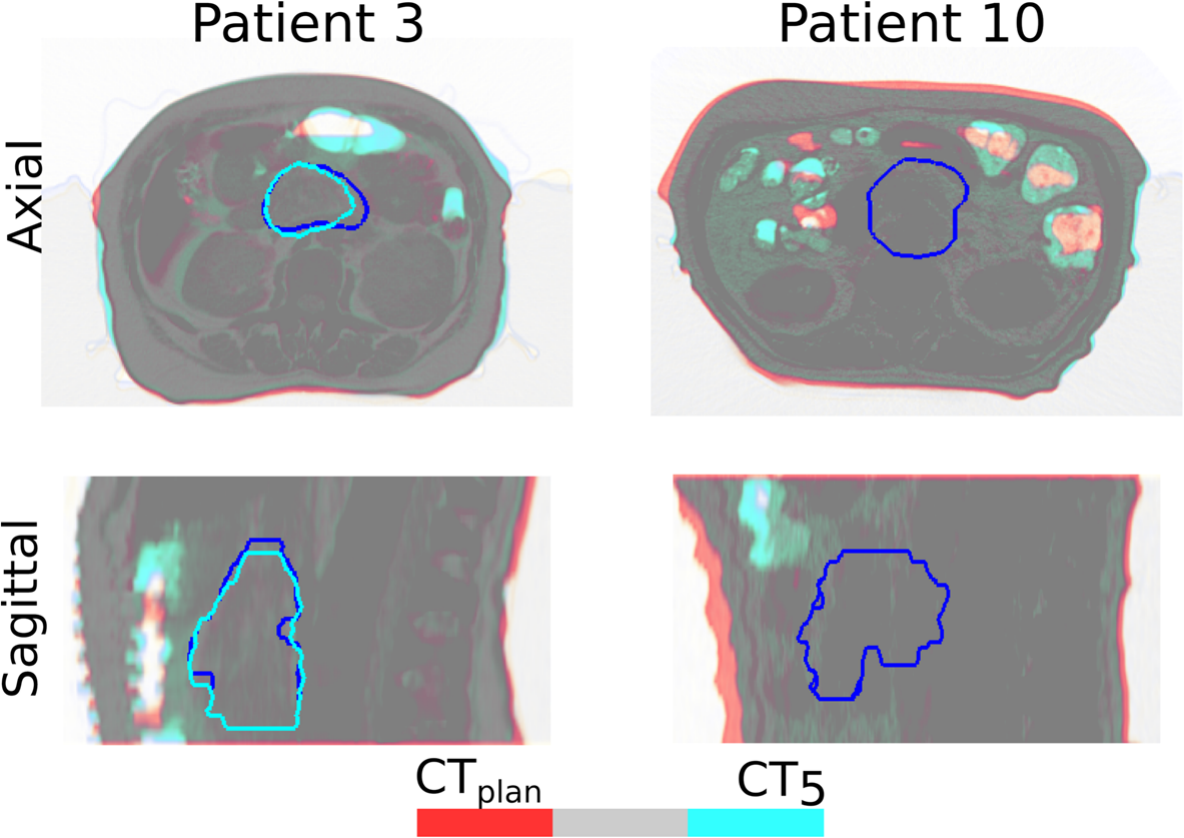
Overlay of the planning CT (*red*) and the CT of the week 5 (*blue*) for the patient 3 and 10. The grey color means perfect overlay between the patient anatomy and the *red/blue* color the region with anatomy changes (i.e. bowel, stomach and weight loss). The *dark blue* contour represents the planning CTV, while the *light blue* the CTV of the week 5 CT
